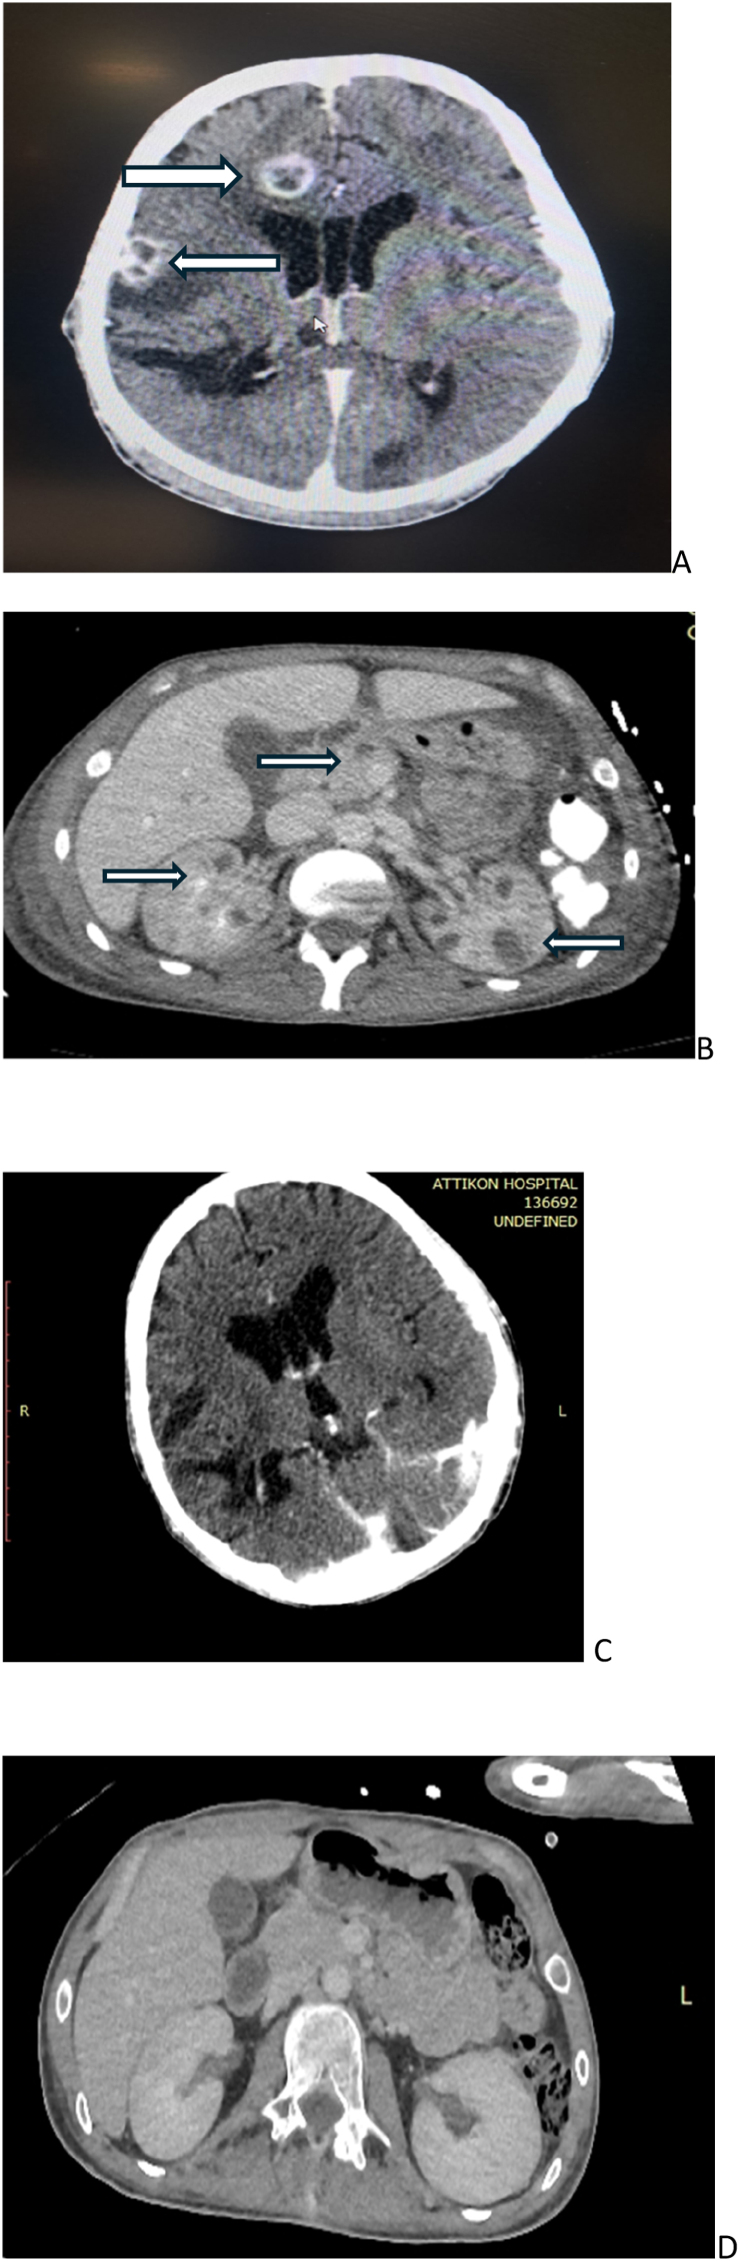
Radiological findings in of cerebrum and abdomen before and after fosmanogepix treatment. A) Abdominal CT imaging prior to antifungal treatment, showing bilateral kidney and pancreatic abscesses B) Brain CT before antifungal treatment, revealing multiple parenchymal hematomas and diffuse abscesses. C) Abdominal CT imaging after 5 months of treatment with fosmanogepix, revealing complete remission of kidney and pancreatic abscesses. D) Brain CT after 5 months of treatment with fosmanogepix, showing progressive improvement.
